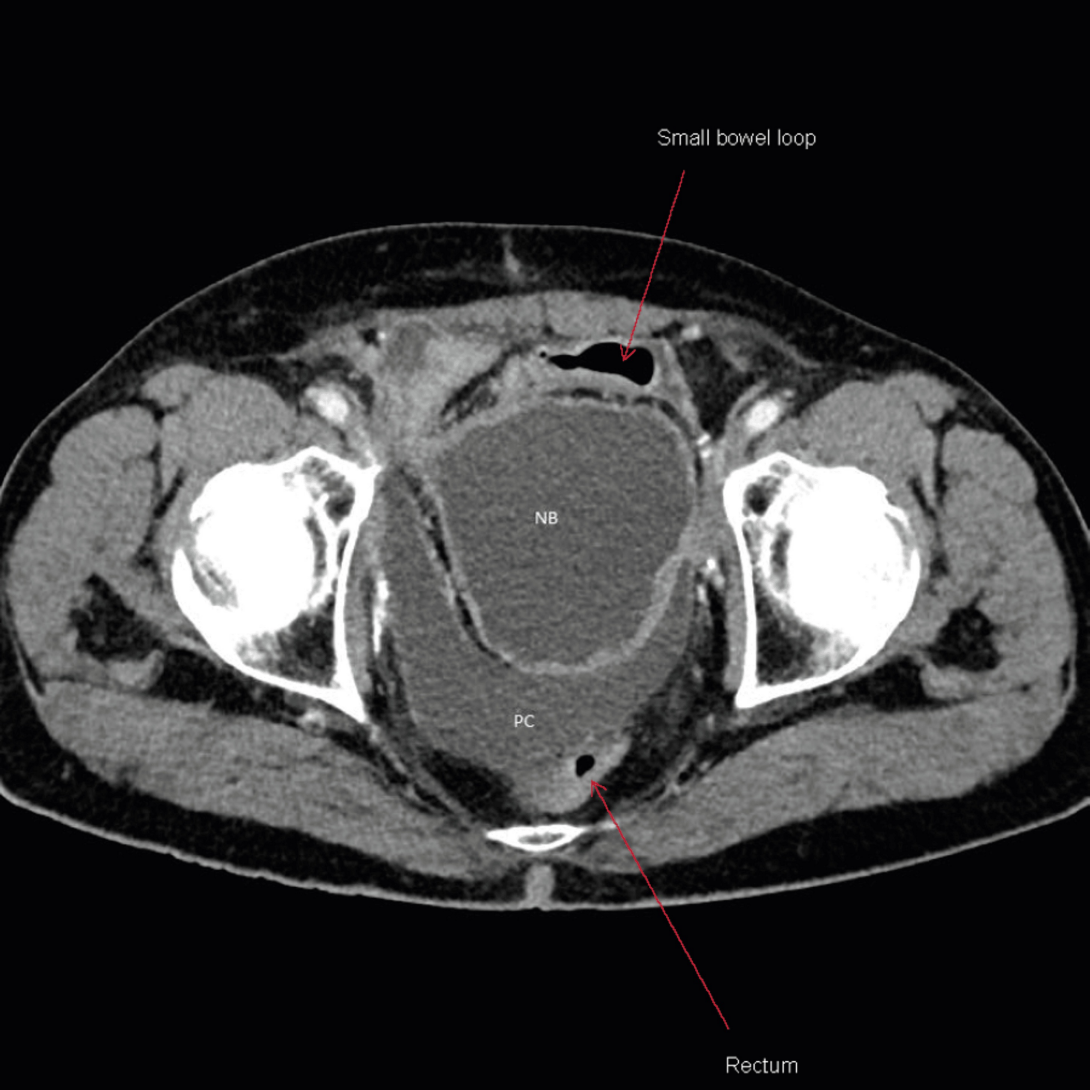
Axial view of contrast-enhanced computed tomography (CECT) performed on the day of admission showing pelvic fluid collection posterior to neobladder NB: Neobladder; PC: pelvic fluid collection
